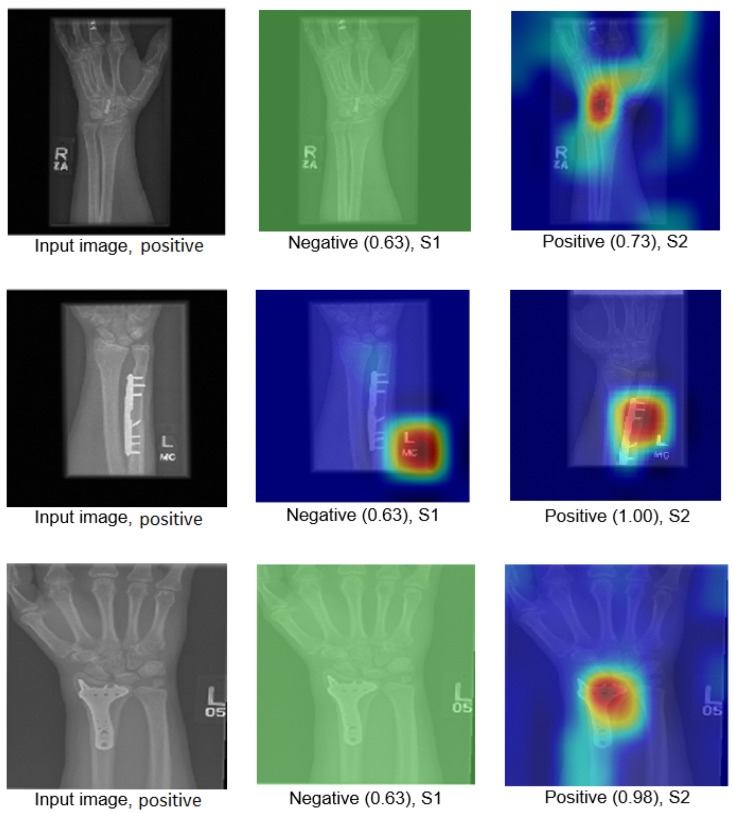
Grad-CAM and score Grad-CAM for the wrist X-ray images. The correct classification is positive.
